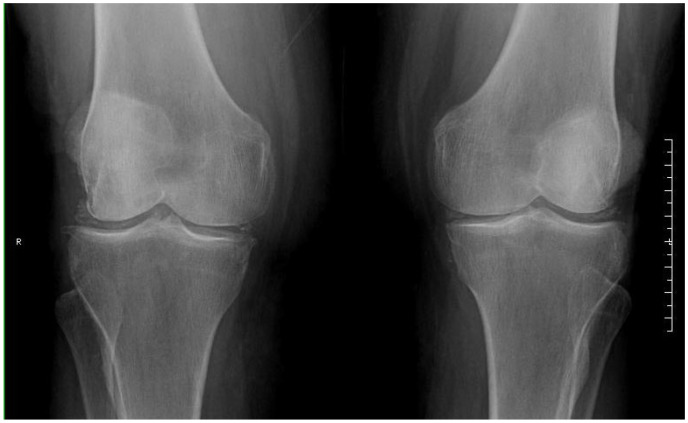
X-ray of the patient’s knees. Osteoarthritis (decreased femorotibial joint spaces and osteophytes) and radiographic chondrocalcinosis.
